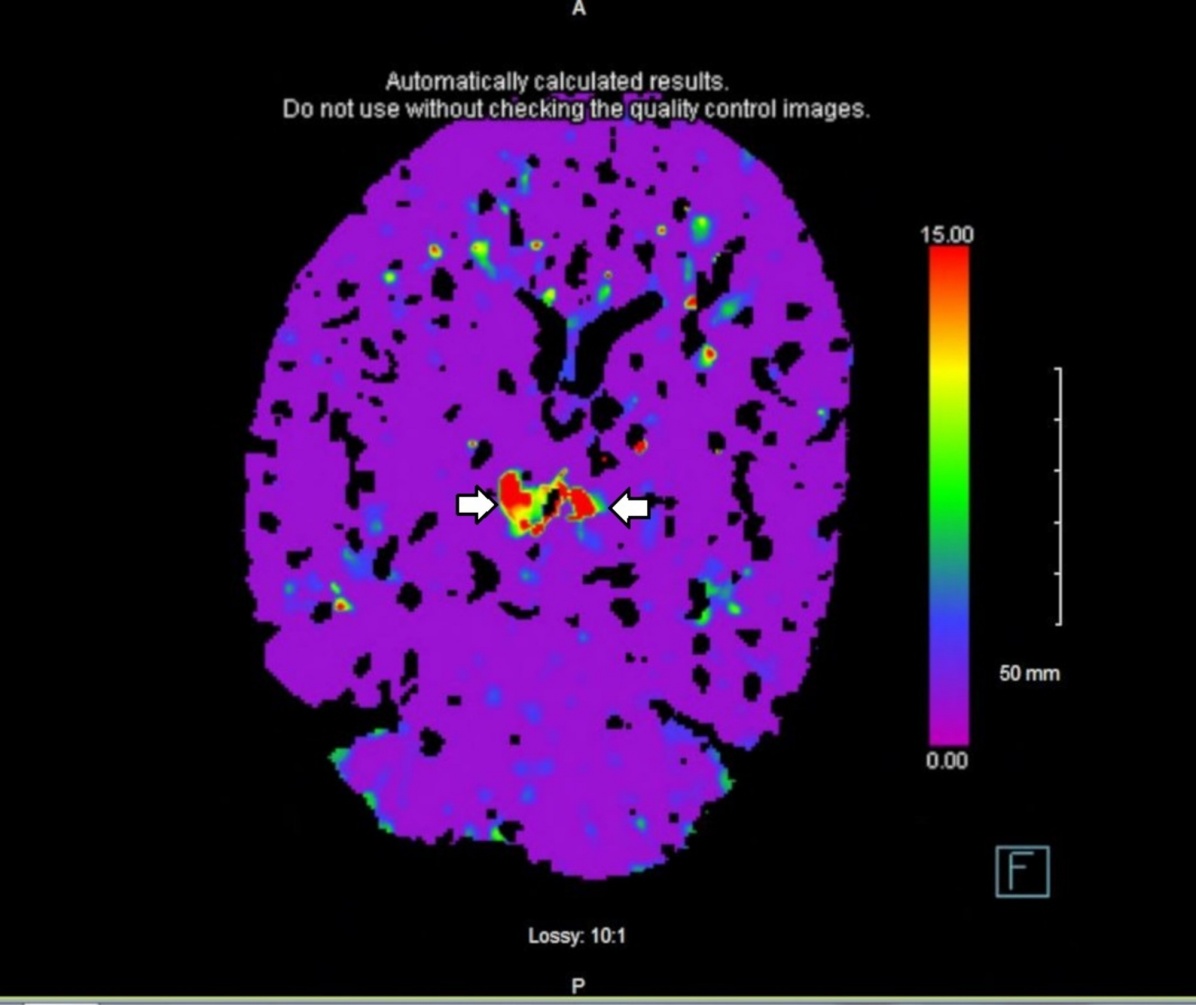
Time to drain CT perfusion source imaging showing decreased perfusion in the midbrain consistent with an AOP stroke. CT, computed tomography; AOP, artery of Percheron.
